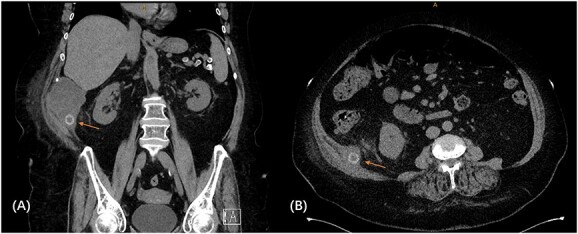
CT scan demonstrating retained gallstone with associated abscess ~62 × 86 × 98 mm in the right flank involving the right posterior transverse abdominis/internal oblique muscle in the coronal (A) and axial (B) views.
